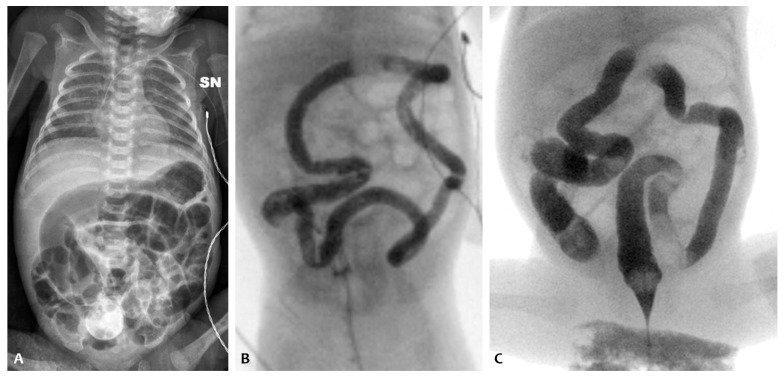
Abdominal X-ray of patient P4 on the 8th day of life (A); contrast enema before the NAC treatment (B) and after the NAC treatment (C).
